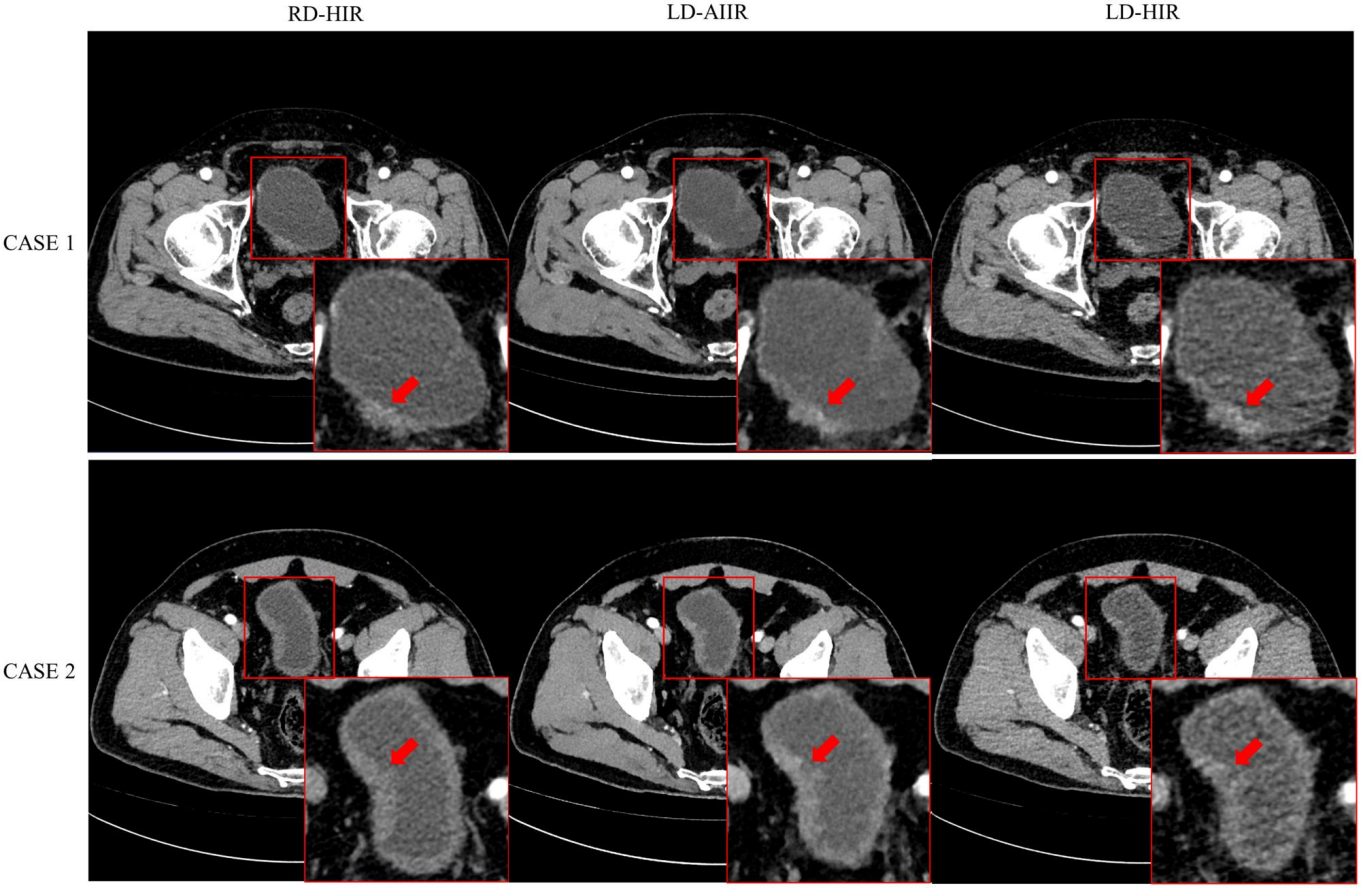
Corticomedullary phase CT images of a 72-year-old man (CASE 1) and a 60-year-old man (CASE 2) at routine-dose with hybrid iterative reconstruction (RD-HIR), low-dose with artificial intelligence iterative reconstruction (LD-AIIR), and with hybrid iterative reconstruction (LD-HIR). Pathology results confirmed both cases were negative for bladder cancer. The diagnostic confidence on RD-HIR, LD-AIIR, and LD-HIR was graded 4, 4 and 4 for CASE 1; and 2, 3, and 4 for CASE 2, respectively, by both radiologists.
